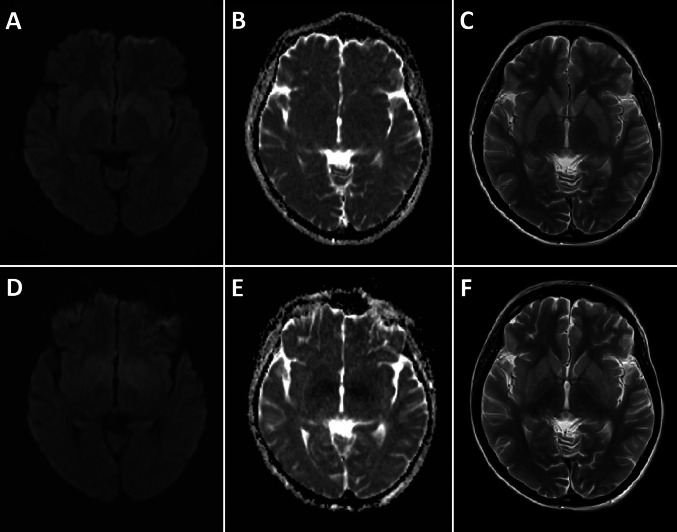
Magnetic resonance imaging (MRI) scans of the brain on day 3; A: Diffusion weighted imaging (DWI), B: apparent diffusion coefficient (ADC), and C: T2 weighted images; all normal. MRI brain scans on day 19; D: DWI, E: ADC, and F: T2 weighted images, showing poorly demarcated areas of restricted diffusion with corresponding ADC hypointensity and T2 hyperintensity in the bilateral basal ganglia and sub-insular regions (including the claustra)
